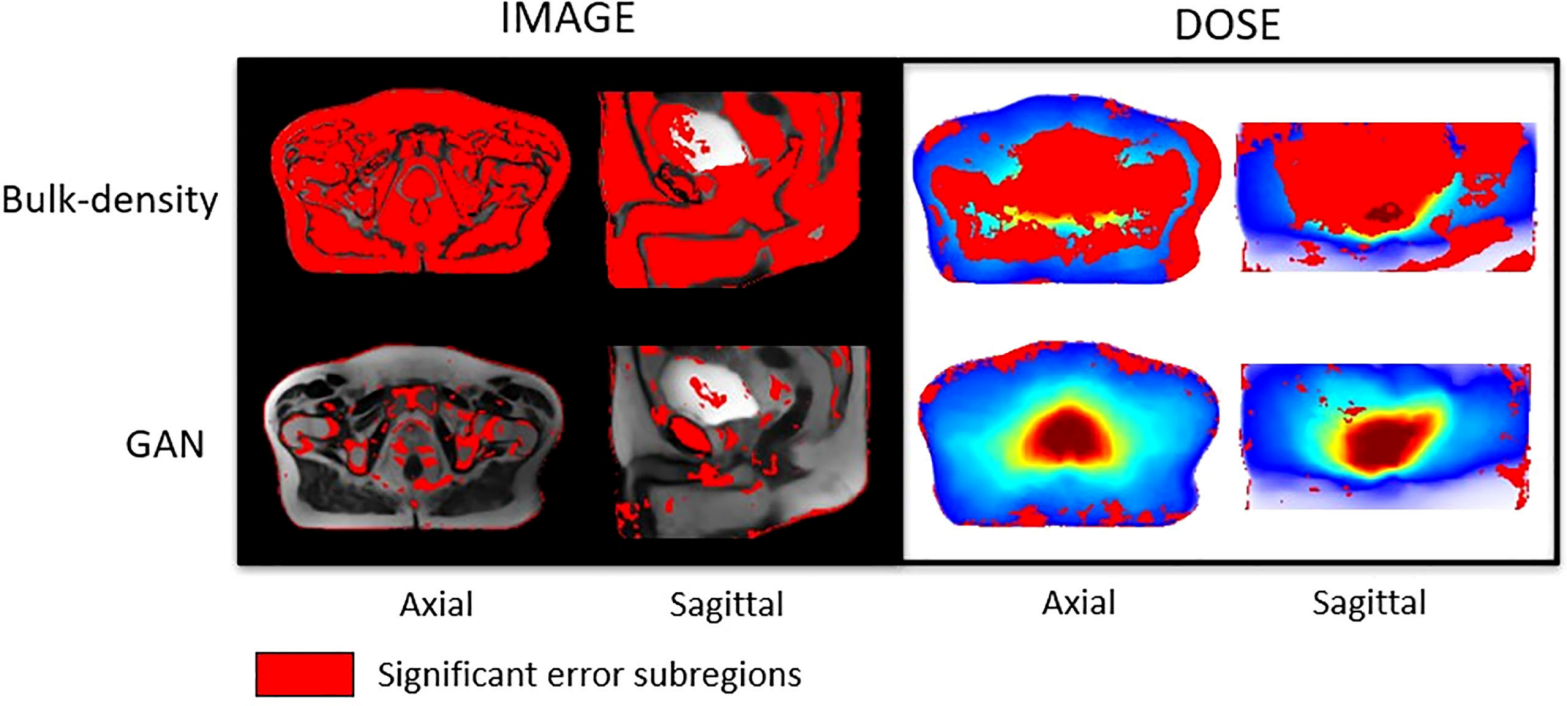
Studentized paired permutation test results. Significant error subregions brought out by Konietschke’s paired permutation test, in red, overlaid on mean MR images in the CCS for HU values (left) and overlaid on the mean dose plans in the CCS for Gy values (right). This statistical test produced p-value maps. Differences of intensities (HU) on one hand, and dose (Gy) on the other hand, were considered as significant for p-value< 0.05.
